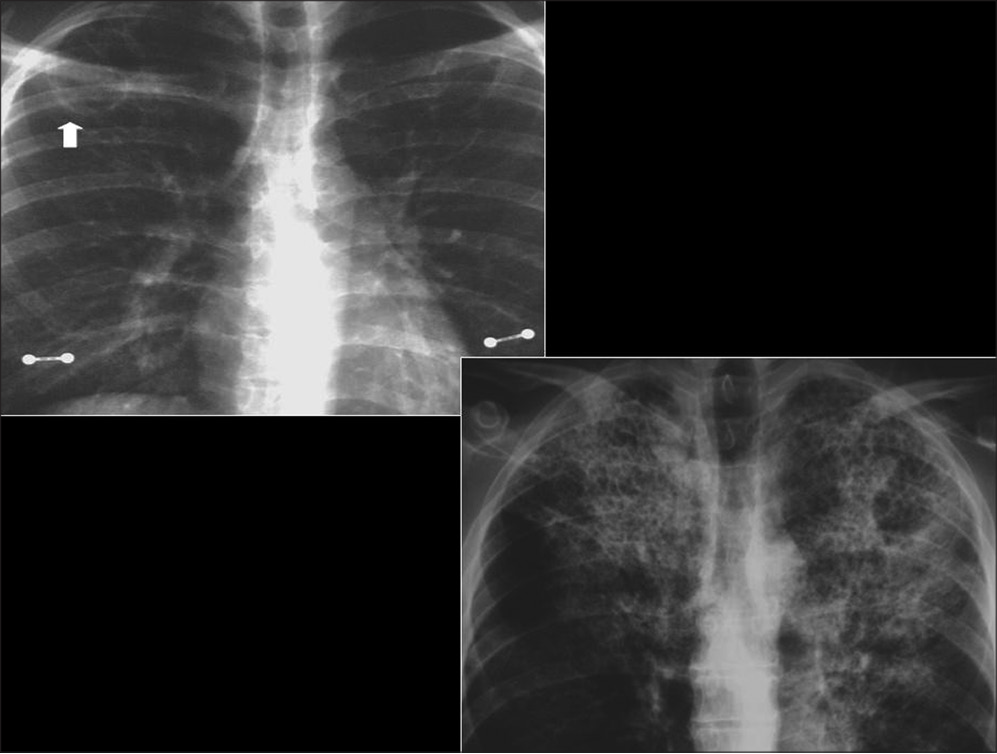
Pneumocystis carinii* pneumonia. Chest X-ray (R) shows a thin-walled cyst in the right upper lobe (arrow). The left image shows multiple cysts in the apical regions related to pentamidine inhalation in a human immunodeficiency virus patient
